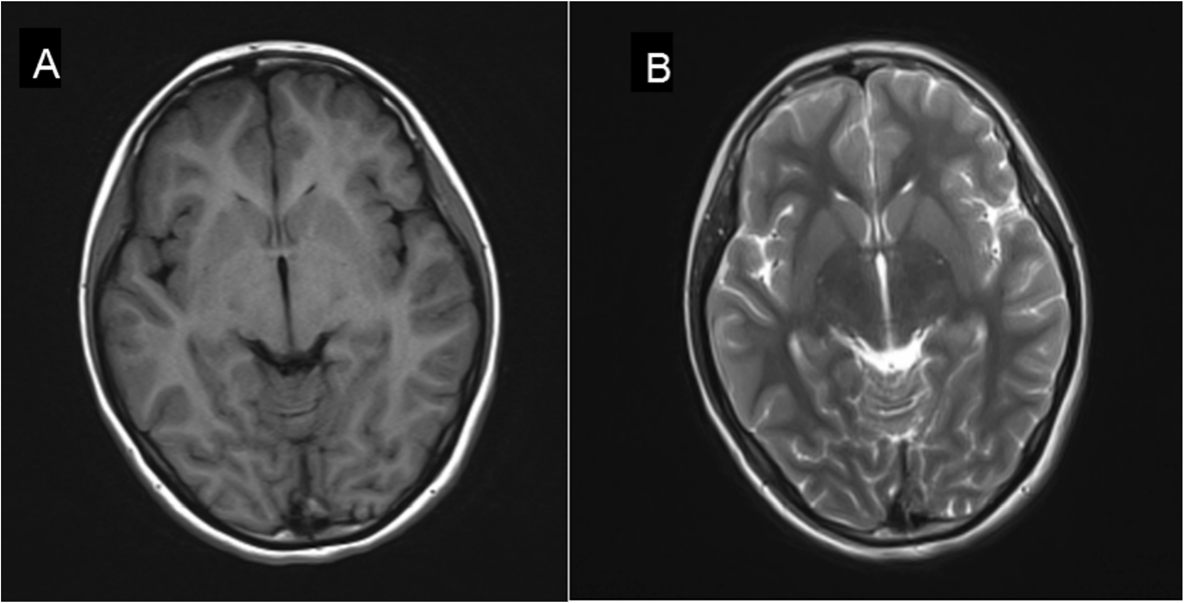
T1-weighted and T2-weighted image showing normal signal intensity in the parenchymal and cerebellum. No abnormally was found in the shape, size and position of ventricle, cistern and sulci
